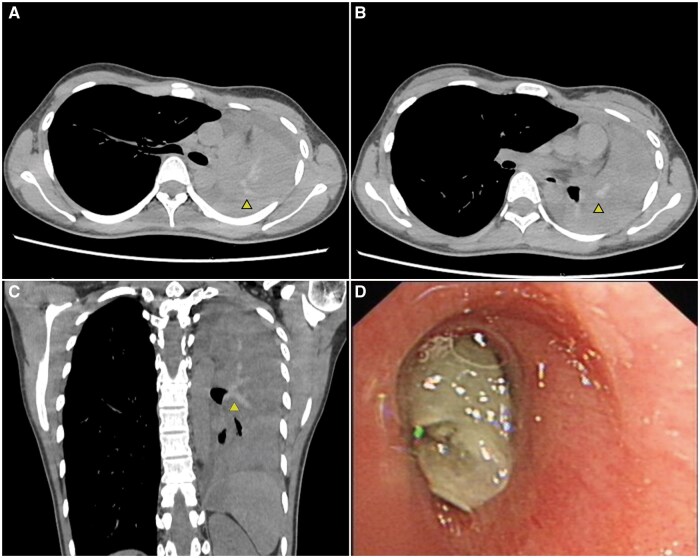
Computed tomography (CT) findings: axial view (A, B) and coronal view (C) showing high-attenuation mucus (arrows) within the left main bronchus and its branches, extending along the tracheal direction, radiating from the left hilum to the periphery with complete atelectasis of the left lung. (D) Flexible bronchoscopy showing a gelatinous mucus plug completely obstructed the left main bronchus.
